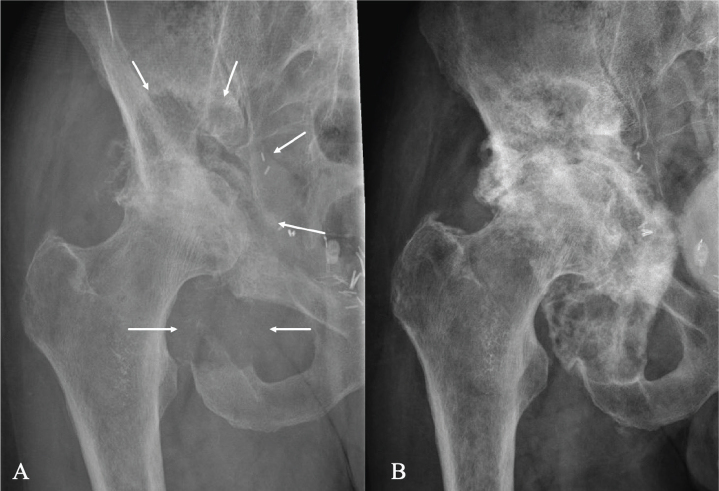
An 82-year-old man with prostate cancer and pathological fractures in the pelvis with protrusion of the femoral head (A). He had severe pain and was unable to bear weight on the right leg. He was treated with denosumab and a single dose of radiotherapy of 8 Gy. Radiography 8 months after the start of treatment revealed increased mineralization (B) and the patient was able to put full weight on his right leg.
